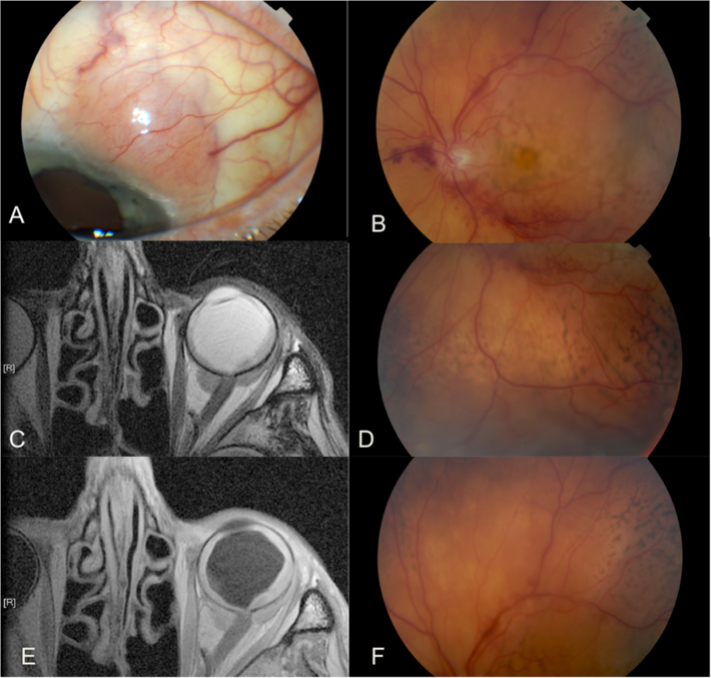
Left eye at presentation. (A) Conjunctival lesion in the superotemporal quadrant, (B) fundus of the left eye showing the posterior pole (B) and choroidal infiltration with secondary retinal detachment in the inferior (D) and superior (F) periphery. (C,E) Magnetic resonance imaging of the orbits revealing left choroidal tumor mass and two lesions around the optic nerve in T1-weighted and T2-weighted images, respectively.
